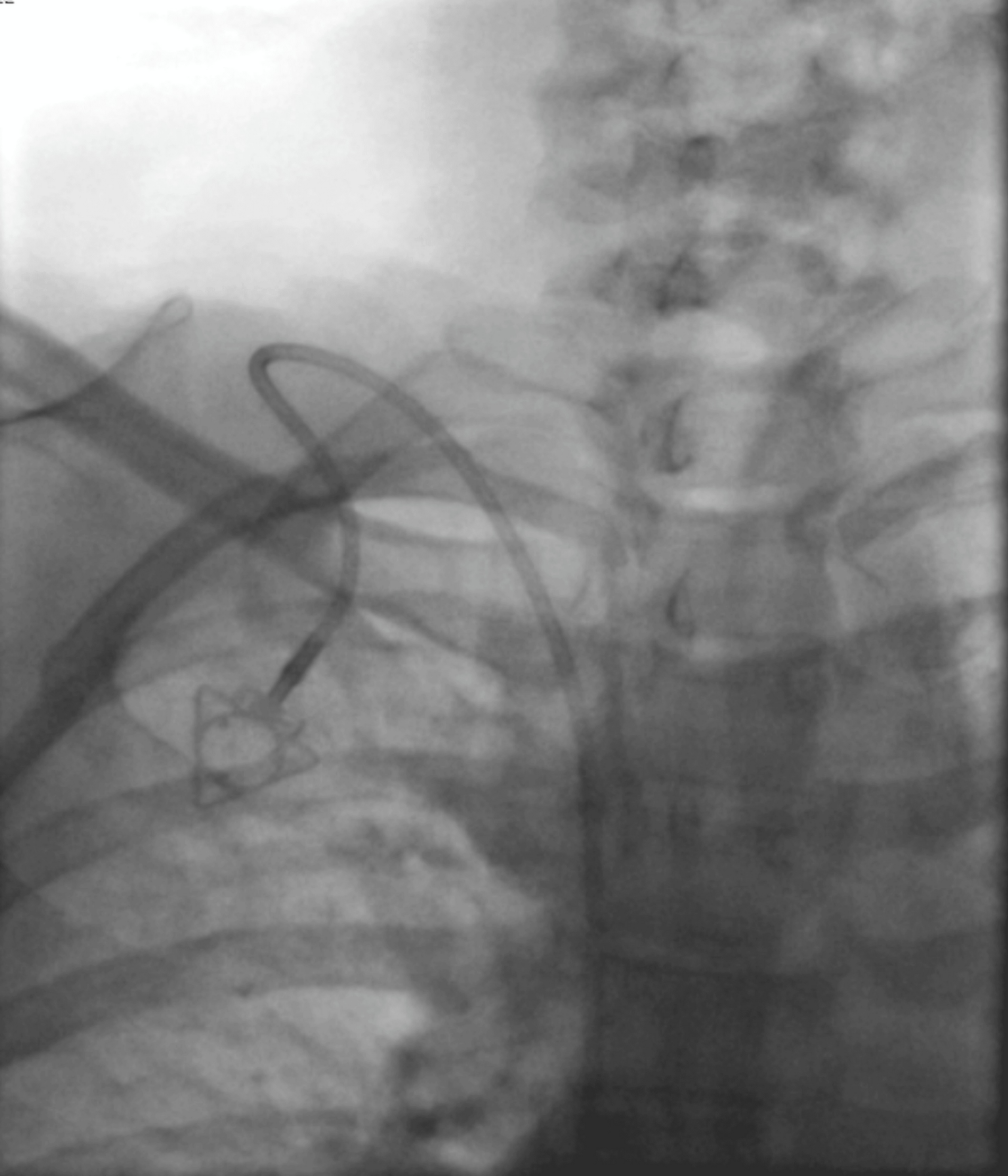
Kinked right catheter Anteroposterior chest radiograph obtained with a C-arm demonstrates a kinked right-sided catheter. This finding may be a consequence of inadequate tunneling. The patient experienced discomfort, and catheter removal was required as a result.
